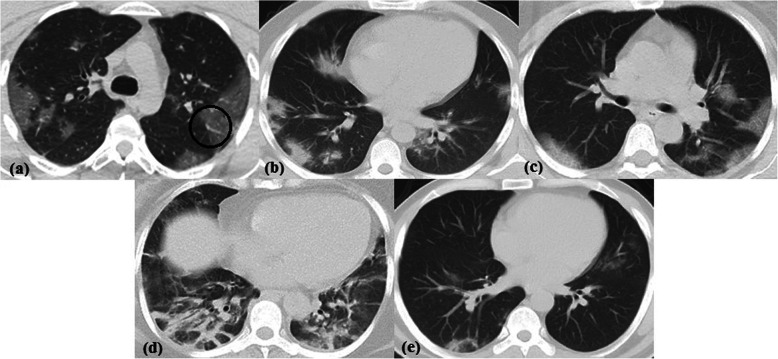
Non-contrast CT chest axial cuts in lung window showing the main findings in RT-PCR proved COVID-19 cases. a Multifocal bilateral peripheral GGO in a 35-year-old male associated with vascular thickening (circle). b Multifocal bilateral peripheral consolidation in a 45-year-old male. c Multifocal bilateral peripheral and central crazy paving in a 55-year-old female. d Peri-lobular fibrosis in a 50-year-old female. e Right lower lung lobe posterior segment reversed halo sign in a 46-year-old male
